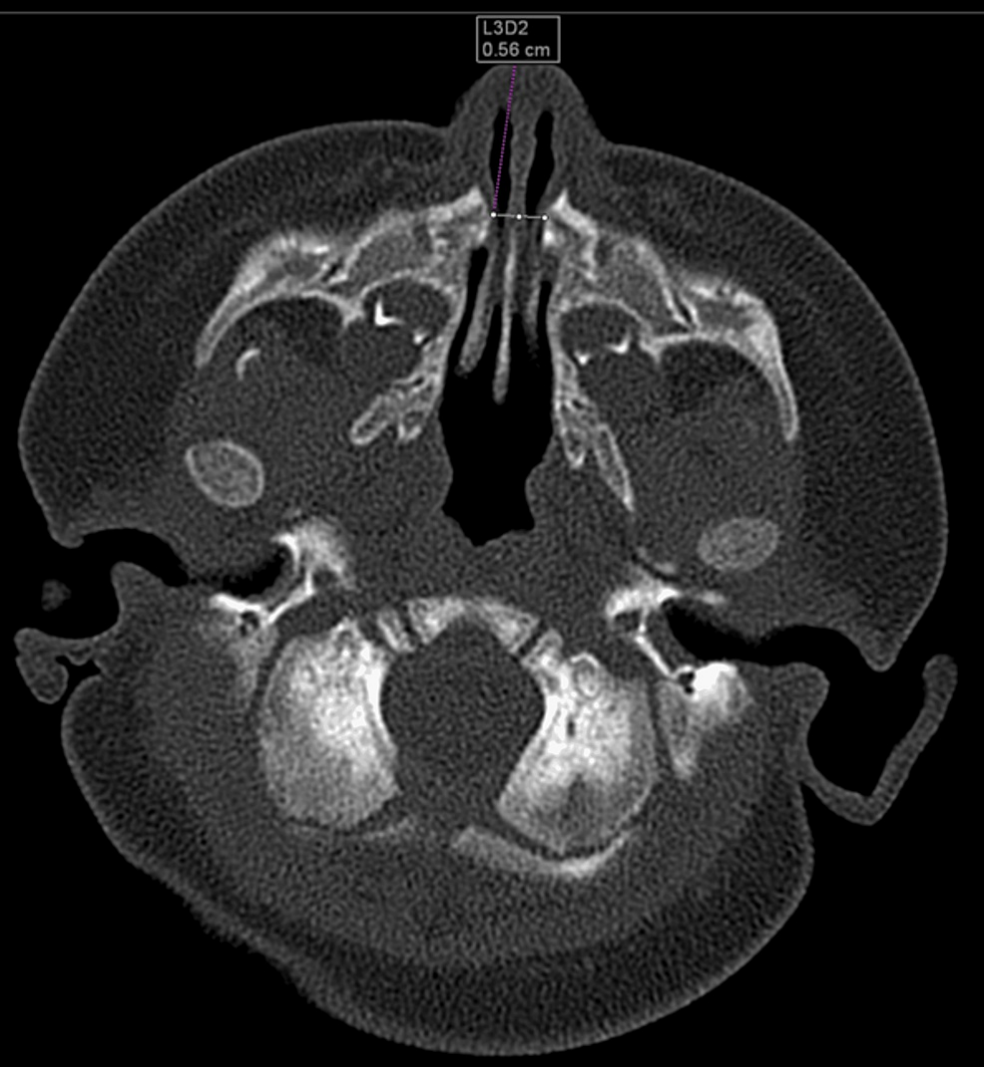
Axial section CT shows a narrow 5.6 mm diameter of pyriform aperture.
